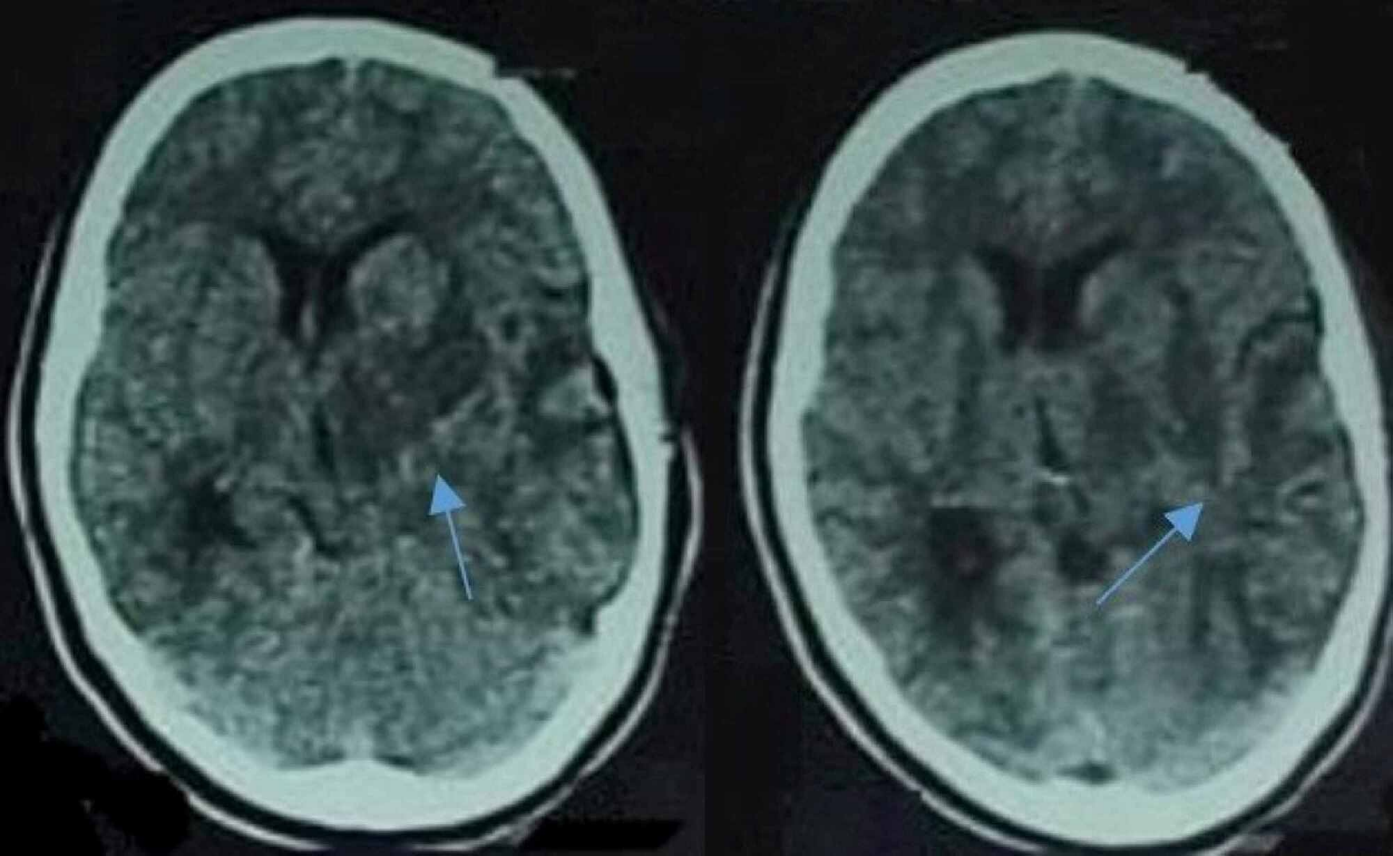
Axial images of CT brain showing significant reduction in abscess size after craniotomy and initiation of antibiotics (blue arrows). CT: Computed tomography
